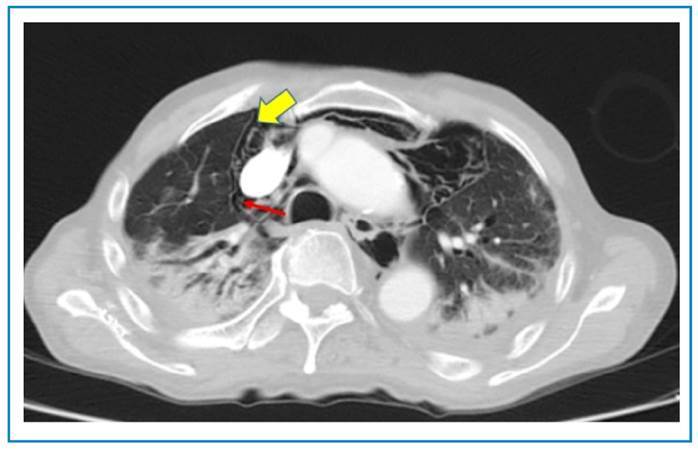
Computed tomography (CT) of the thorax with a small right apical pneumothorax, shown by a yellow arrow. The red arrow shows a bronchopleural fistula.
